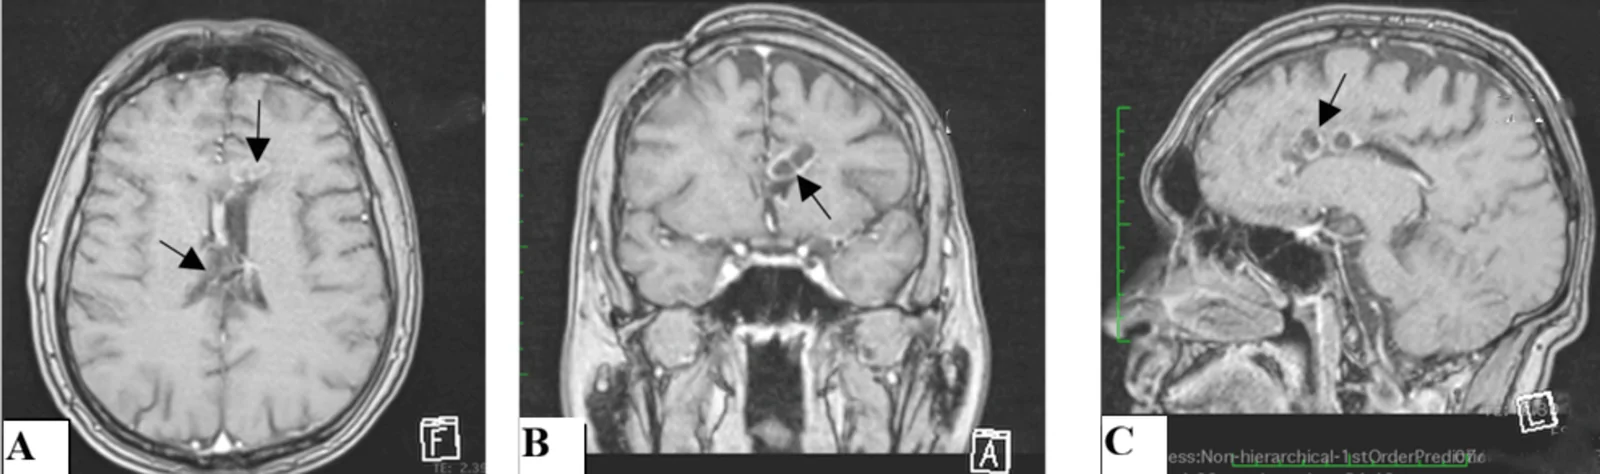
MRI findings (A) Axial T1-weighted contrast-enhanced brain MRI. A midline 87 lesion involving the ventricular wall is observed, showing an irregular morphology with 88 heterogeneous gadolinium enhancement (black arrows). (B) Coronal T1-weighted contrast-enhanced 89 brain MRI (black arrow). (C) Sagittal T1-weighted contrast-enhanced brain MRI (black arrow) MRI: magnetic resonance imaging
